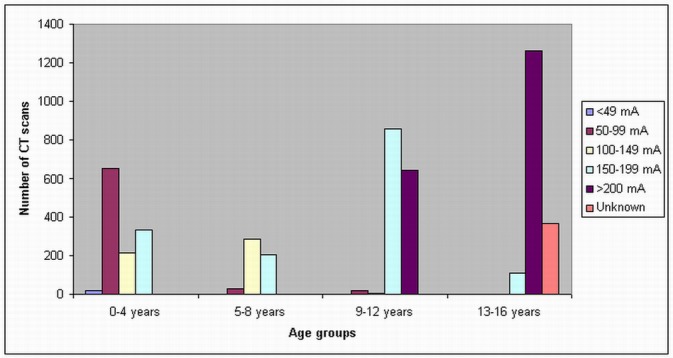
Tube current settings used for paediatric head, chest and abdominal CT scans among three hospitals. High tube current is used with increasing age of patients, however, a tube current of 150–199 mA was still used in a number of CT scans in the patients less than 5 years old.
